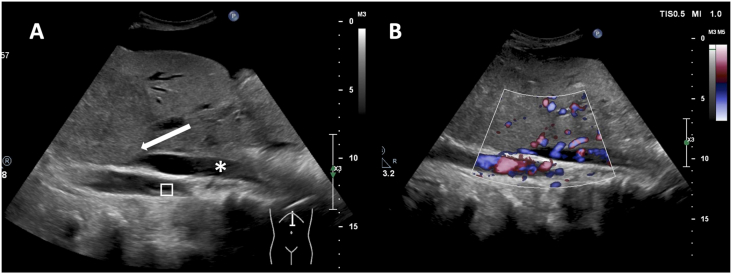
Abdominal ultrasonography findings. (A) A large mass is compressing the inferior vena cava (white arrow). * inferior vena cava, abdominal aorta. (B) A color doppler image showed congestion of blood flow in the inferior vena cava. . (For interpretation of the references to color in this figure legend, the reader is referred to the Web version of this article.)
